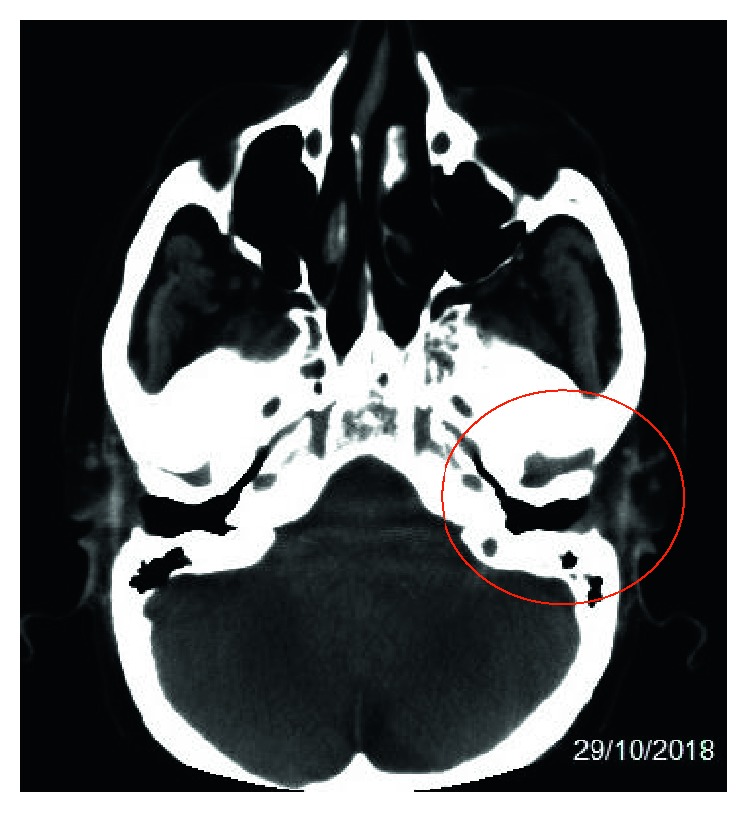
CT scan of the left ear without contrast.
